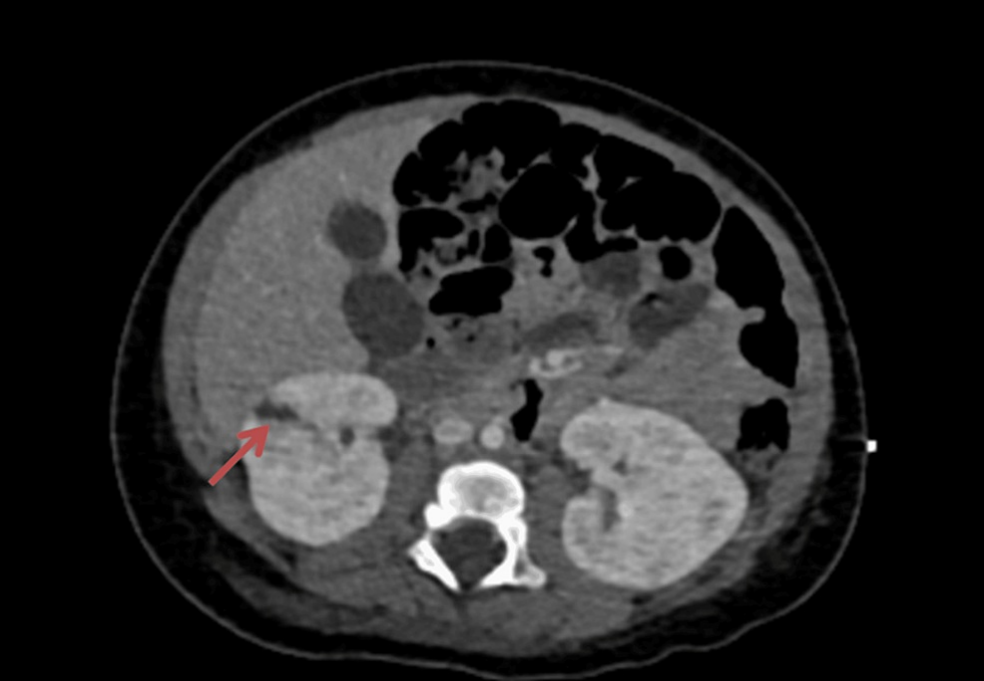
Contrast-enhanced computed tomography of the abdomen shows a small peripheral right renal wedge-shaped infarction (arrow).
